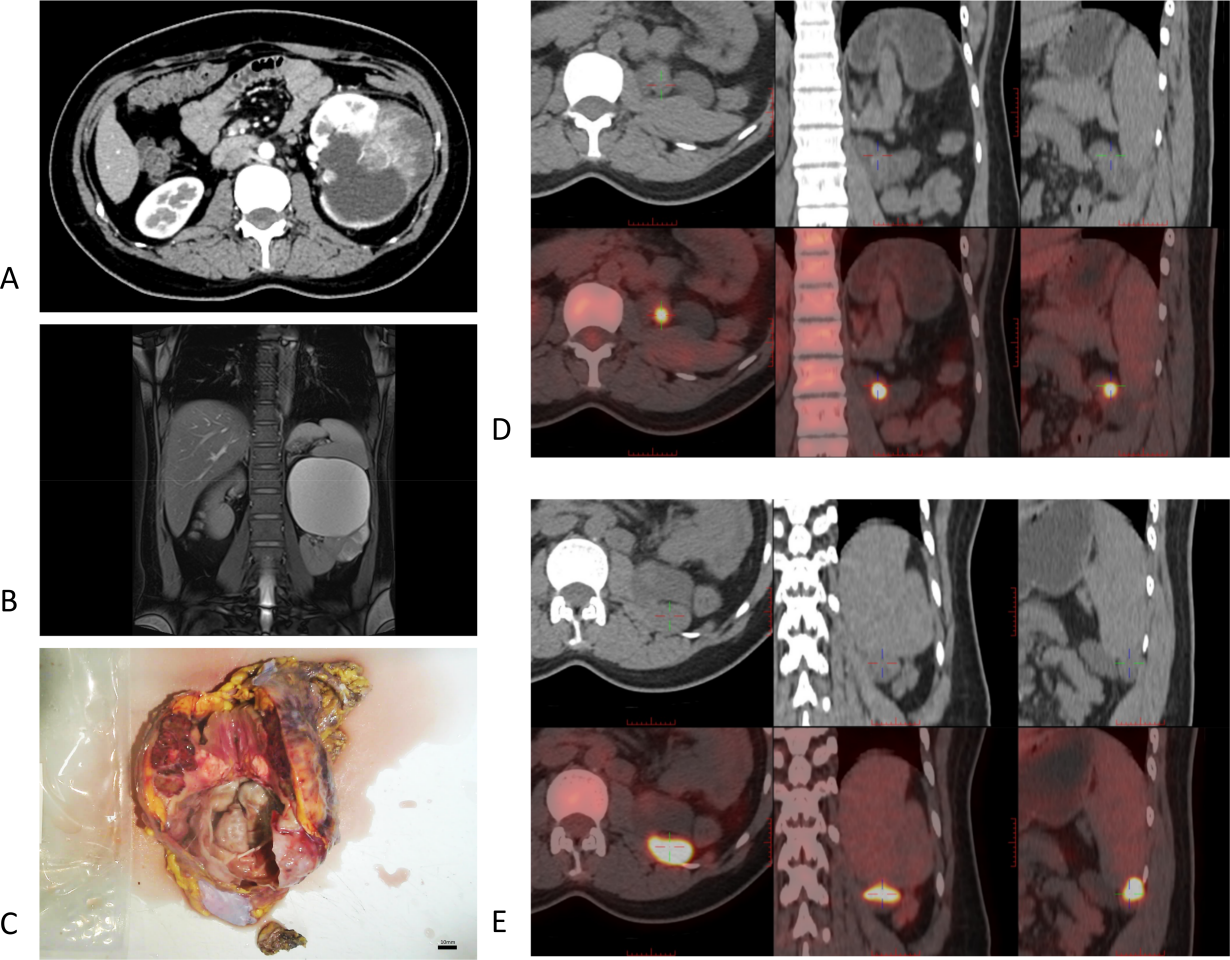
Imaging and gross findings of Case 5. (A) The solid components of the tumor showed mild post-contrast enhancement. (B) On MRI, the left renal lower pole showed a slightly hypointense signal on T1WI and a slightly hyperintense, heterogeneous signal on T2WI. A prominent T1-weighted contrast-enhanced signal was observed, suggesting possible intratumoral hemorrhage. (C) Gross pathology: The kidney was completely dissected with easily separated capsule and fat. The cut surface revealed gray-red to gray-brown masses in the renal pole, with indistinct borders from the surrounding parenchyma. The tumor appeared to invade the renal sinus but not the renal pelvis. (D, E) Recurrence on PET-CT: PECT-CT examination showed recurrence: a small amount of fluid accumulation in the left nephrotomy area and multiple occupancy, with increased FDG uptake; the size of the adjacent peritoneum was approximately 2.6 × 1.7 cm, the SUVmax was approximately 10.1, and the size of the renal area after surgery was 4.5 × 3.7 cm, and the SUVmax was approximately 12.
